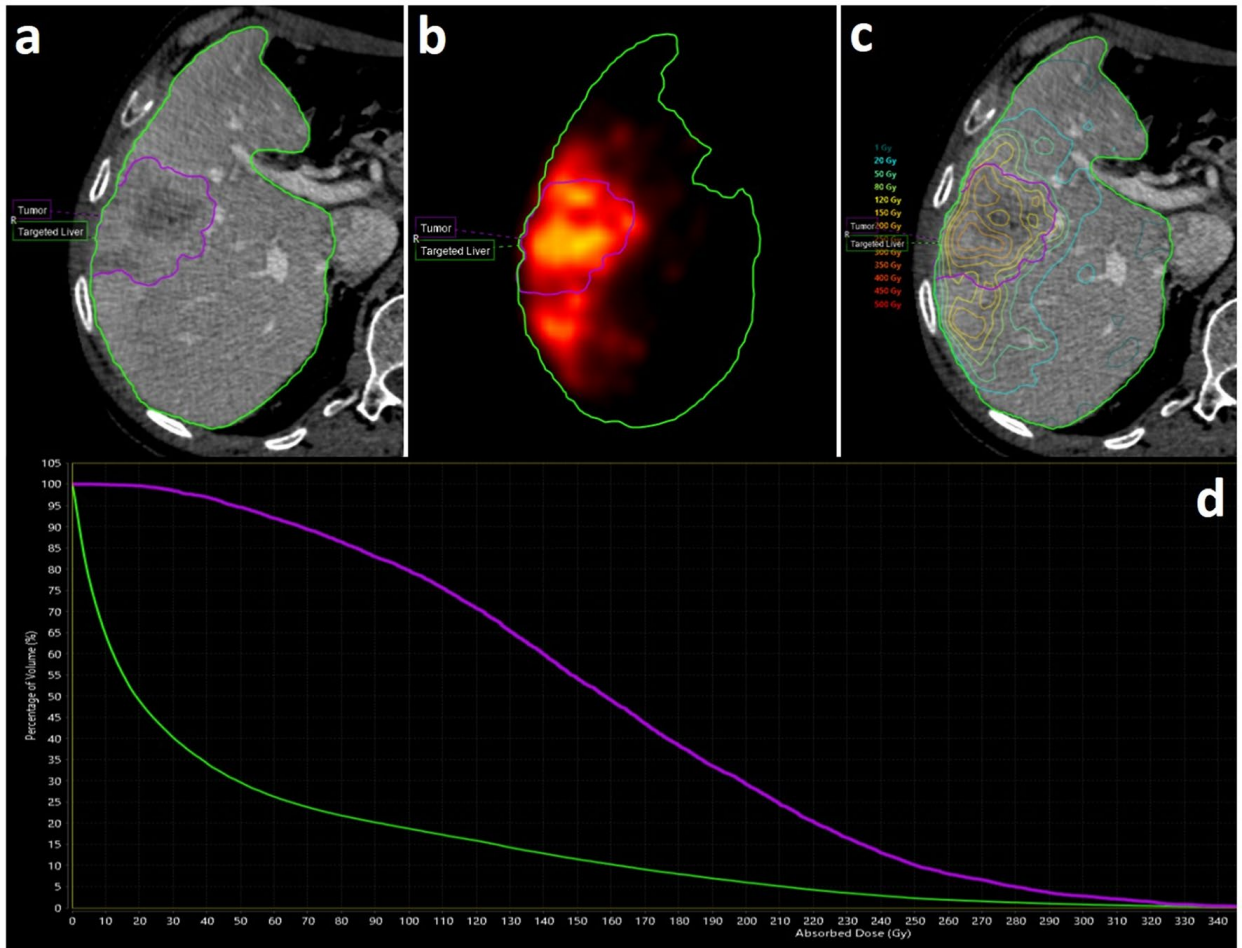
A representation of imaging processing by using the dosimetry software (Simplicit90Y, Mirada Medical, Oxford, UK). On upper left panel (a), VOIs semi-automatically defined on the contrast-enhanced CT over the targeted liver and tumor. On upper central panel (b), the same VOIs transferred over the previously co-registered ^90^Y-PET. On upper right panel (c), calculated isodose distribution within the targeted liver and tumor. On bottom panel (d), dose-volume histograms (DVH) of the targeted liver and tumor.
